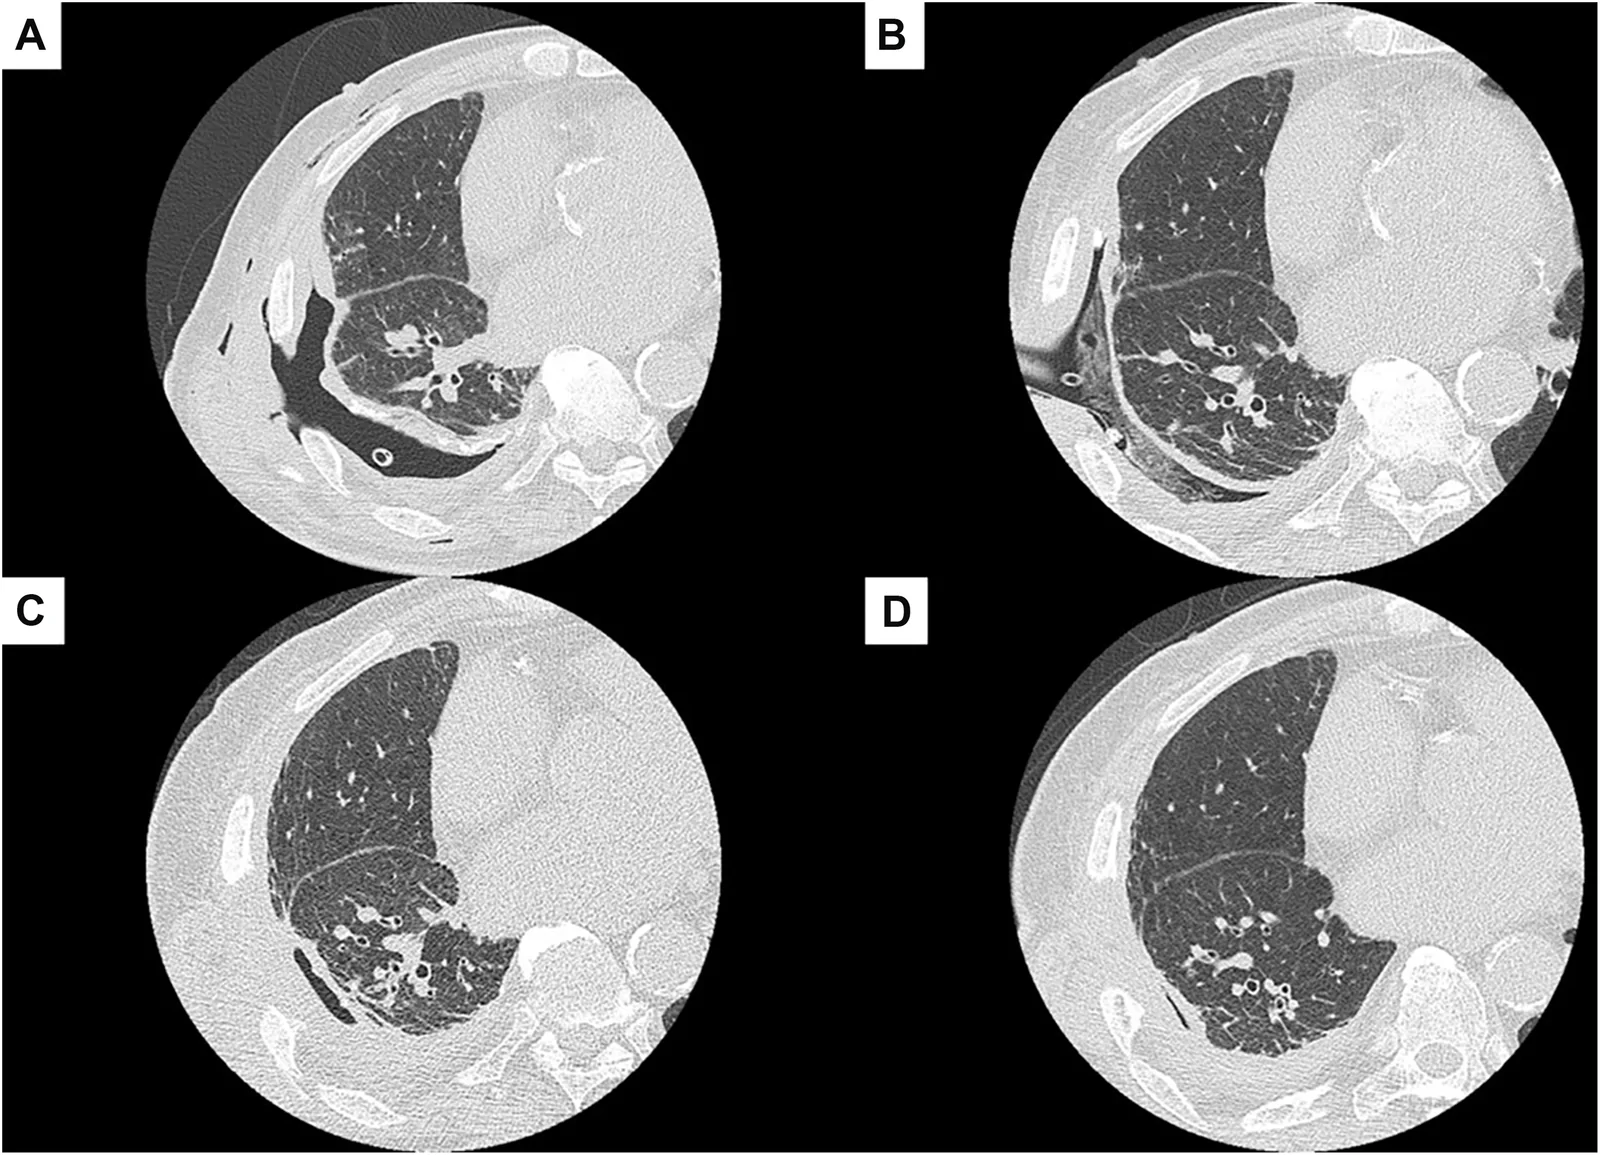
Computed tomography imaging shows the course of a new negative pressure wound therapy (NPWT) using suction tubes after open-window thoracostomy. (A) Compared with before introducing the new NPWT method, (B) marked improvement in lung expansion and thickening of the parietal pleura was observed 22 days after introducing the new NPWT method. (C) A reduction in dead space was observed on day 98. (D) By day 166, the dead space had nearly disappeared, achieving closure of the empyema cavity.
